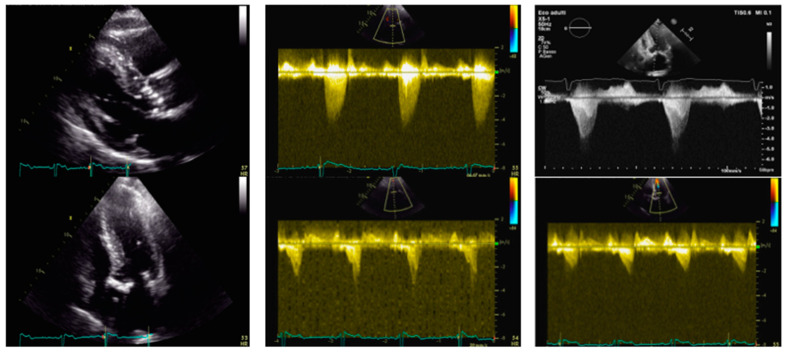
Transthoracic echocardiography of an 80-year-old man before and after aortic valve percutaneous replacement and pacemaker implantation. Left panel. PLAX view (upper) and 4-chamber view (lower). IVS telediastolic diameter = 18 mm; LVEDVi = 54 mL/m^2^. Central panel. Aortic valve peak velocity of 4.5 m/s (upper) with a mean gradient of 50 mmHg and dynamic LVOT gradient (lower) of 45 mmHg after the Valsalva maneuver. Right panel after TAVI. Increased dynamic LVOT gradient after TAVI (upper) of 65 mmHg after the Valsalva maneuver, which is reduced after pacemaker implantation (lower). IVS: interventricular septum. LVEDVi: left ventricular end-diastolic volume indexed. LVOT: left ventricular outflow tract. TAVI: transcatheter aortic valve implantation.
